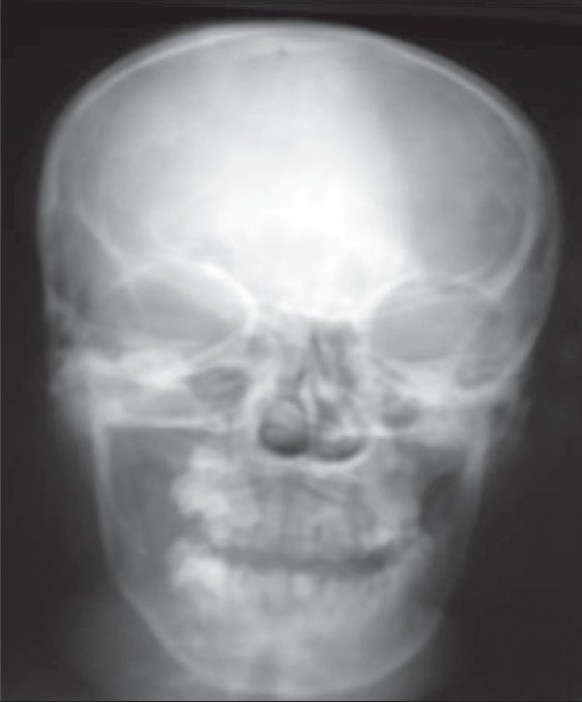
Plain frontal radiograph shows sclerosis of the right frontal and zygomatic bones
